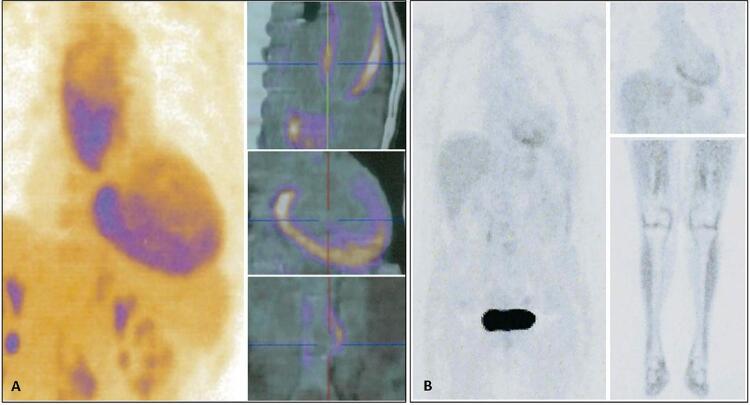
Painel A – PET-CT (Fluorodesoxiglicose) com alta atividade metabólica na aorta ascendente e infrarrenal (*). Ligeira atividade difusa no miocárdio (ponta de seta). Este estudo de imagem também foi notável pelo metabolismo anormal nas carótidas e no eixo arterial dos membros inferiores (possivelmente explicando a sensação de calor no pescoço e as mialgias). Painel B – PET-CT de corpo inteiro (Fluorodesoxiglicose) após um ano de tratamento: resolução completa com atividade metabólica normal no miocárdio, aorta ascendente e infrarrenal, carótidas e eixo dos membros inferiores.
